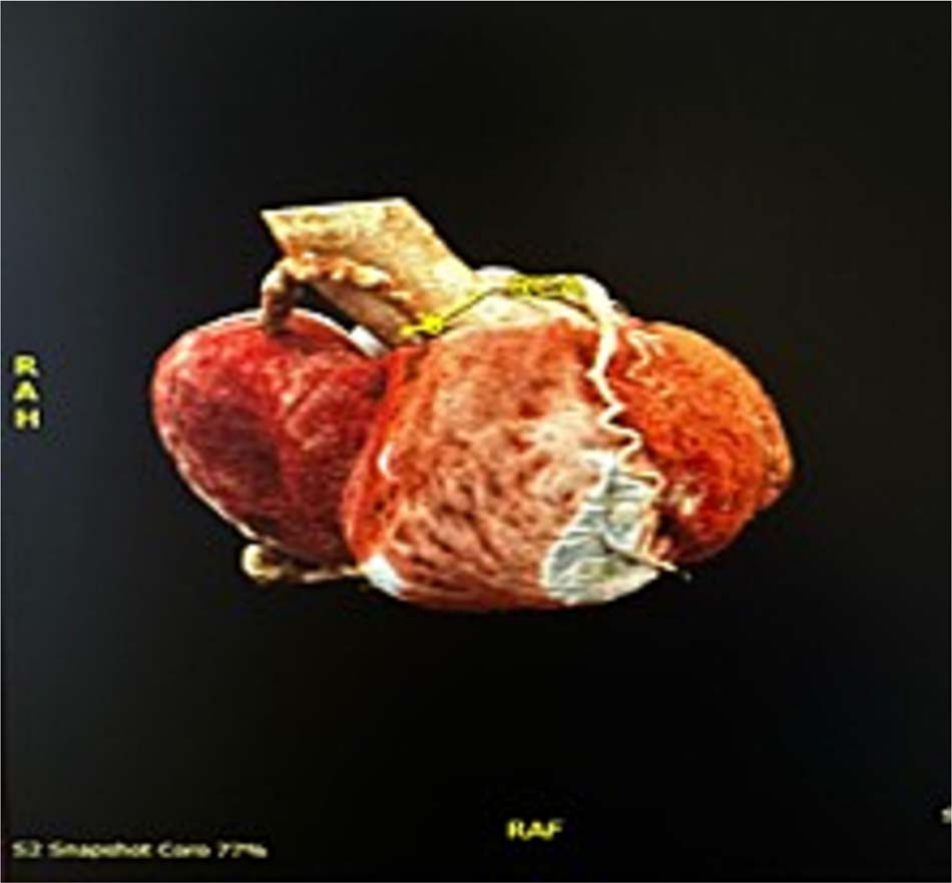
3D reconstruction from CT thorax showing the right coronary aneurysm compressing right heart.
